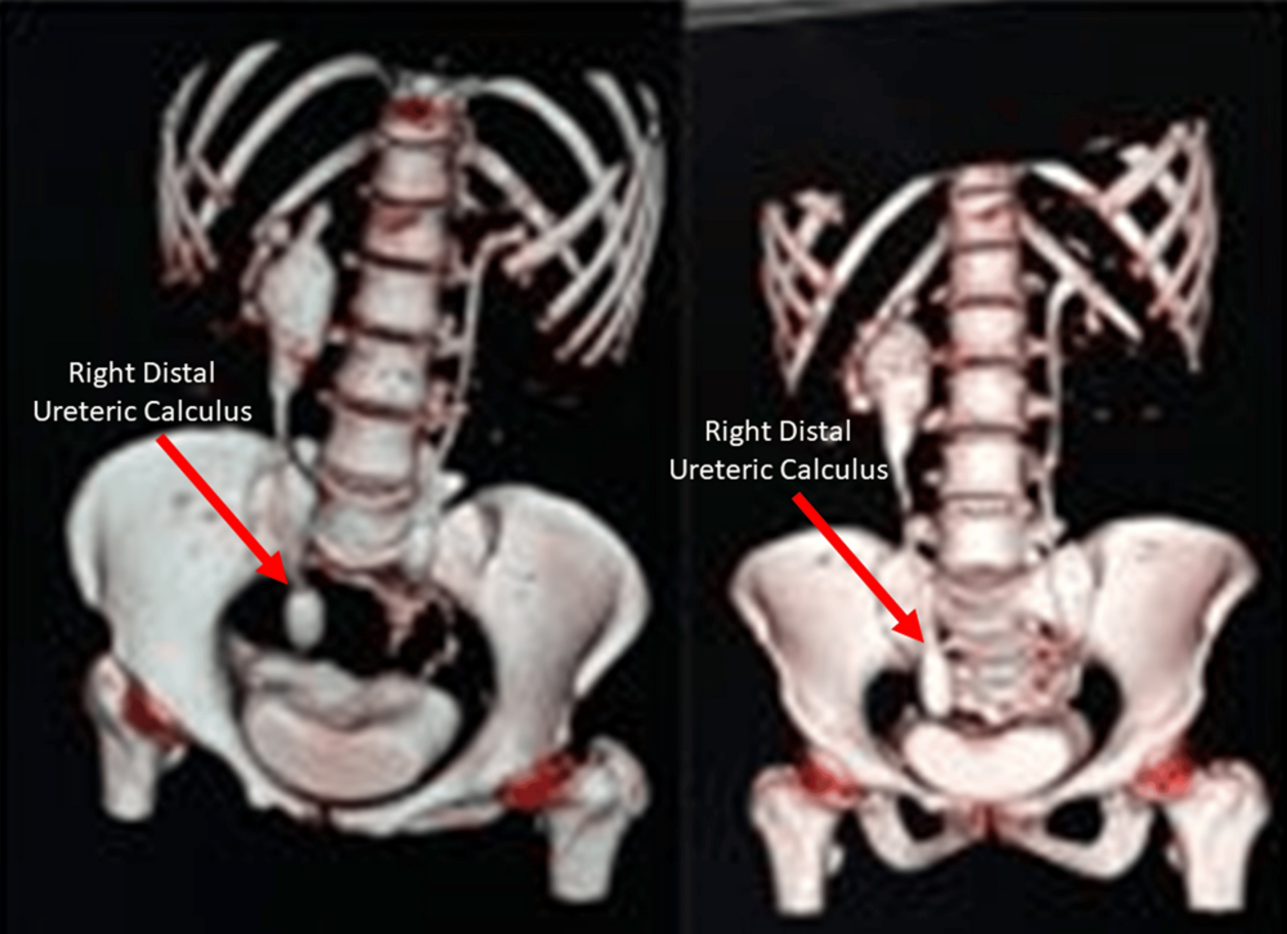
CT scan pelvis with 3D reconstruction image showing right distal ureteric calculus
